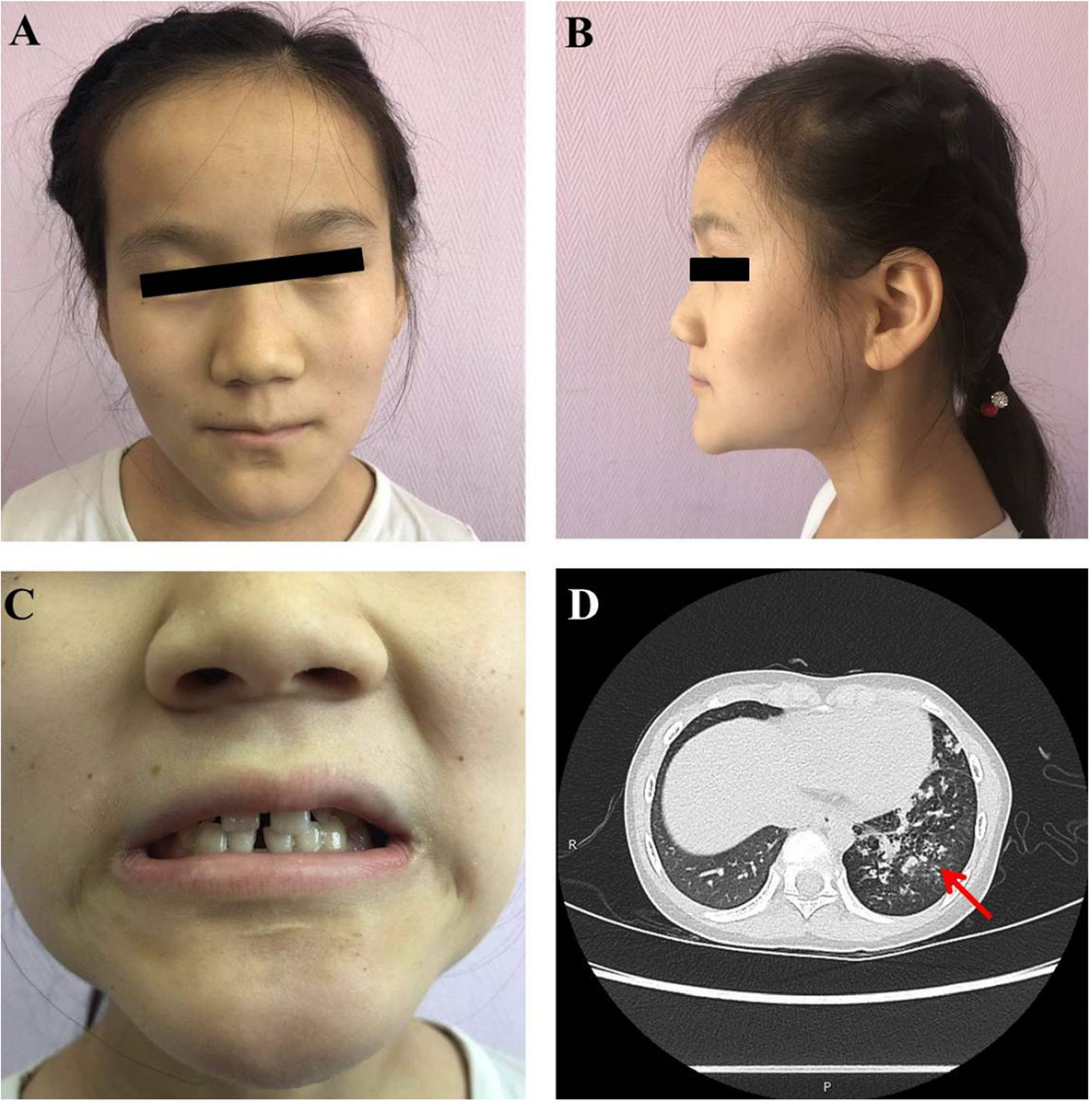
Phenotype of the patient. a-b This patient did not have characteristic facial feathers of 14q11-q22 deletion syndrome. c The patient had normal primary dentition and agenesis of permanent teeth. d Thoracic CT showed interstitial and parenchymal lesions and bronchiectasis in both lungs (red arrow)
